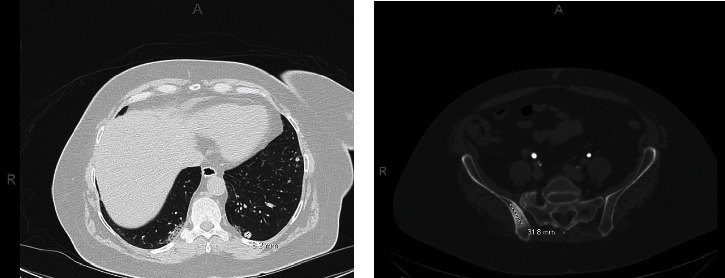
(a) A follow-up CT of the abdomen/pelvis revealed multiple new and enlarging pulmonary nodules in bilateral lungs, and a new sclerotic lesion in the right ilium (b).
